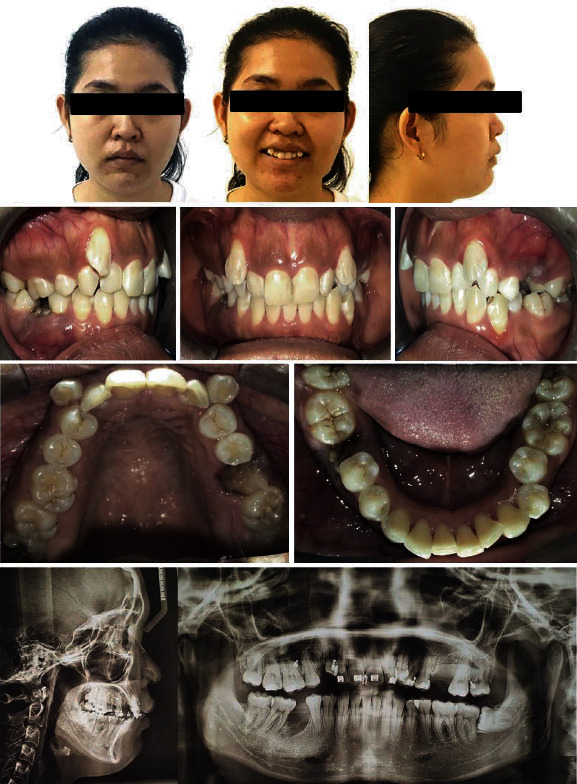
Pretreatment records with radiographs.
